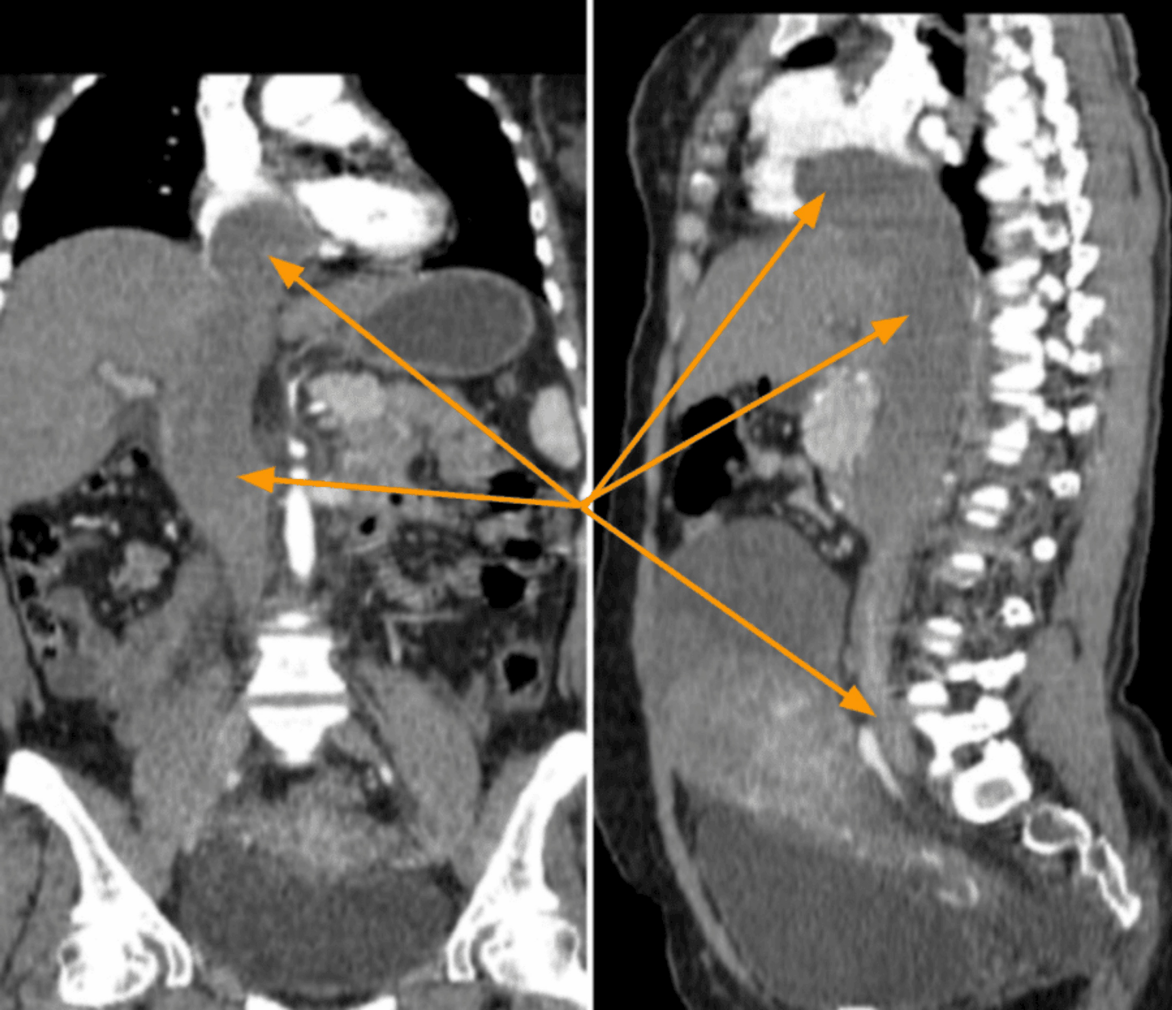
Coronal and sagittal reformatted contrast-enhanced CT images Images showed the pelvic mass invading the right gonadal vein and tracking superiorly into the dilated inferior vena cava (IVC), extending up to the right atrium and protruding into the right ventricle (indicated by the arrows).
